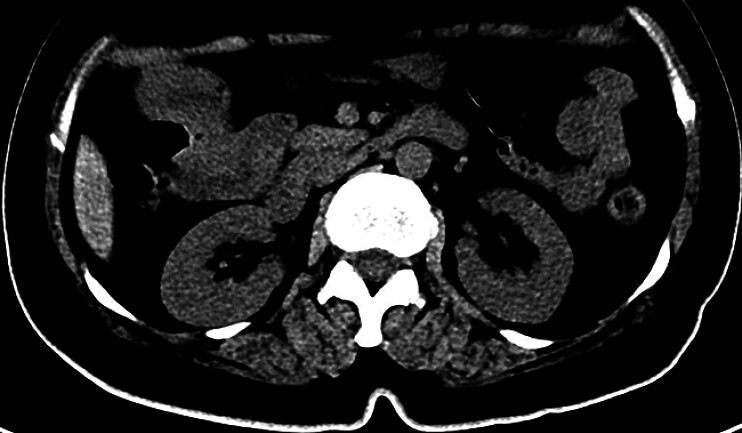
Follow-up computed tomography scan did not show any signs of jejunal obstruction.
